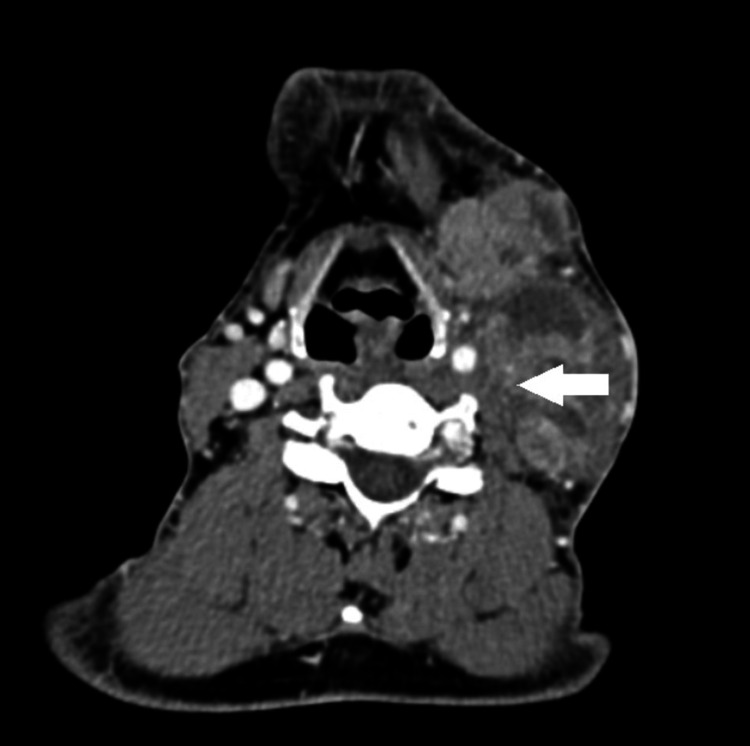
Computed tomography image of the neck (axial view) Computed tomography image of the neck, axial cut in venous phase showing encasement of the internal jugular vein.
